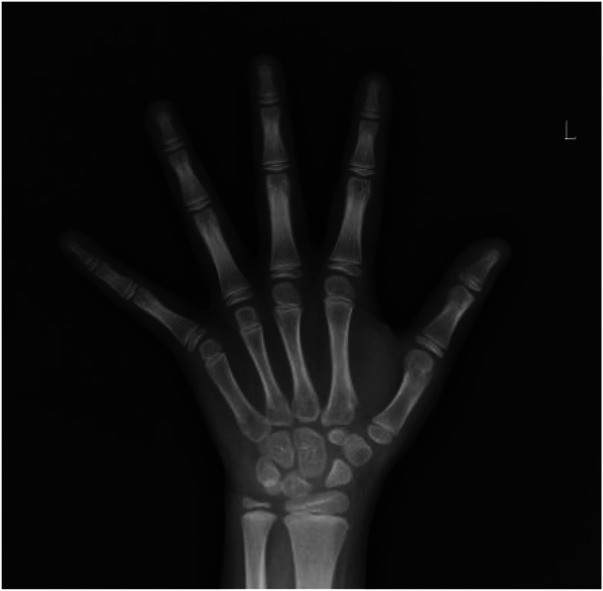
Routine bone age radiograph (bone age assessment at 4 weeks post-treatment indicates bone age equivalent to approximately 10 years of age in females).
